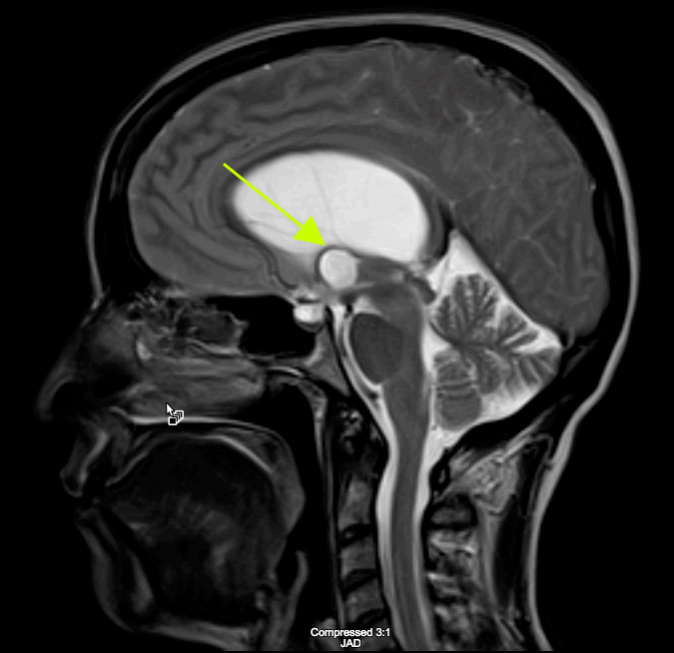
MRI of the Brain without Contrast A: T2 weighted sagittal image demonstrating a colloid cyst measuring 1.3 cm by 1.3 cm at the level of the Foramen of Monro.
